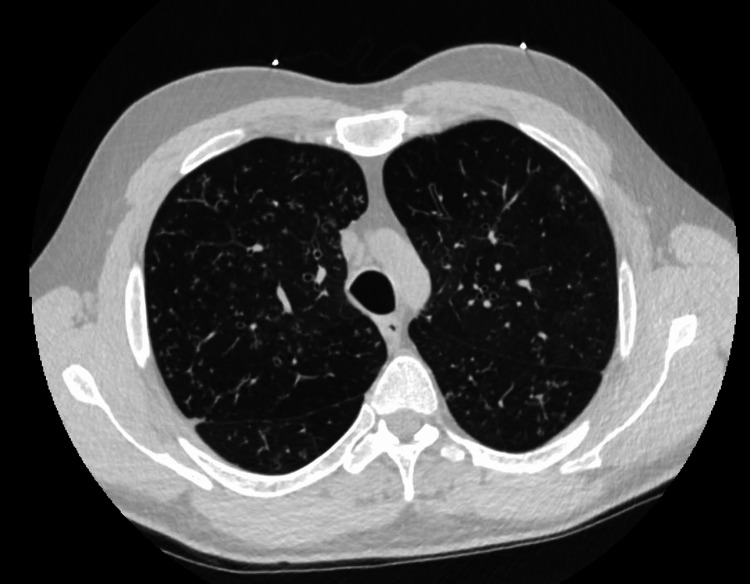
CT scan of the chest showing air trapping and tree-in-bud opacities Tree in-bud opacities and bronchiectasis of the airways
